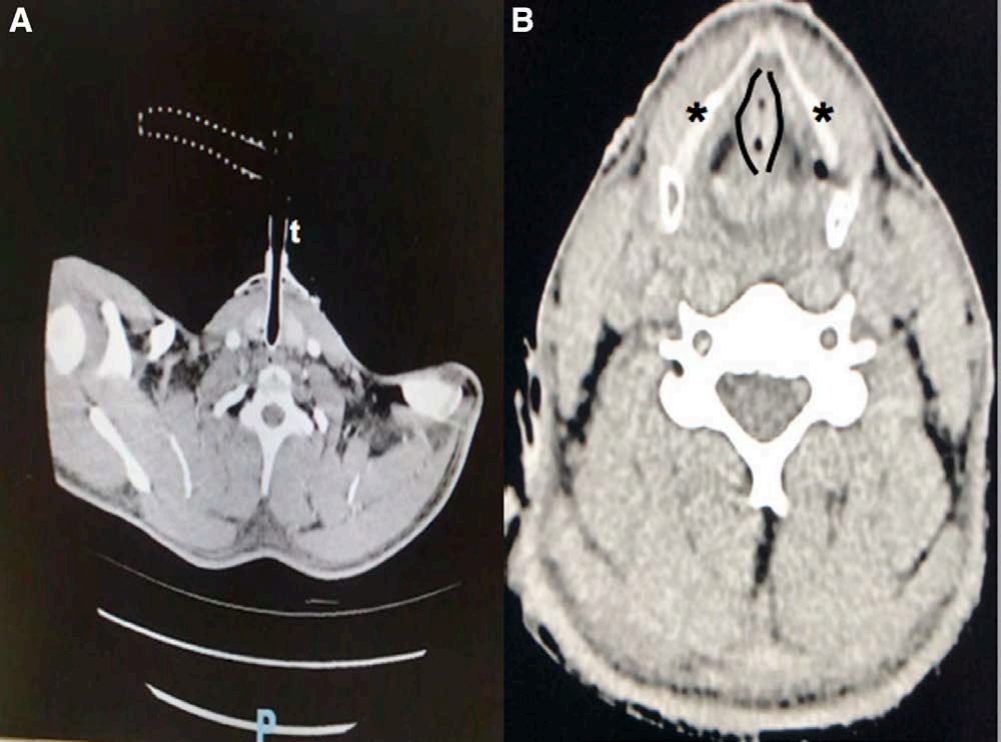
Computed tomography scan. A, Correct placement of the tube (t) within the lumen of the trachea. B, Hypopharynx and oropharynx full-thickness obstruction with a complete narrowing of the supraglottic space: edema of vocal cords (black lines) and soft tissue behind the thyroid cartilage (black stars).
